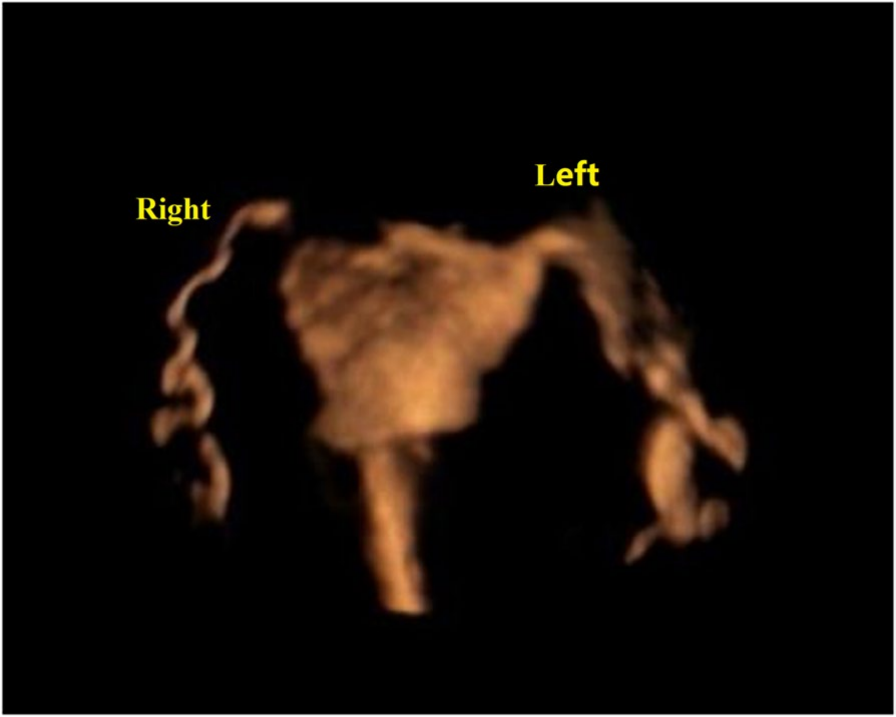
Ultrasonography showing nodular thickening or a walking curl or inversion in the local part of the fallopian tube
